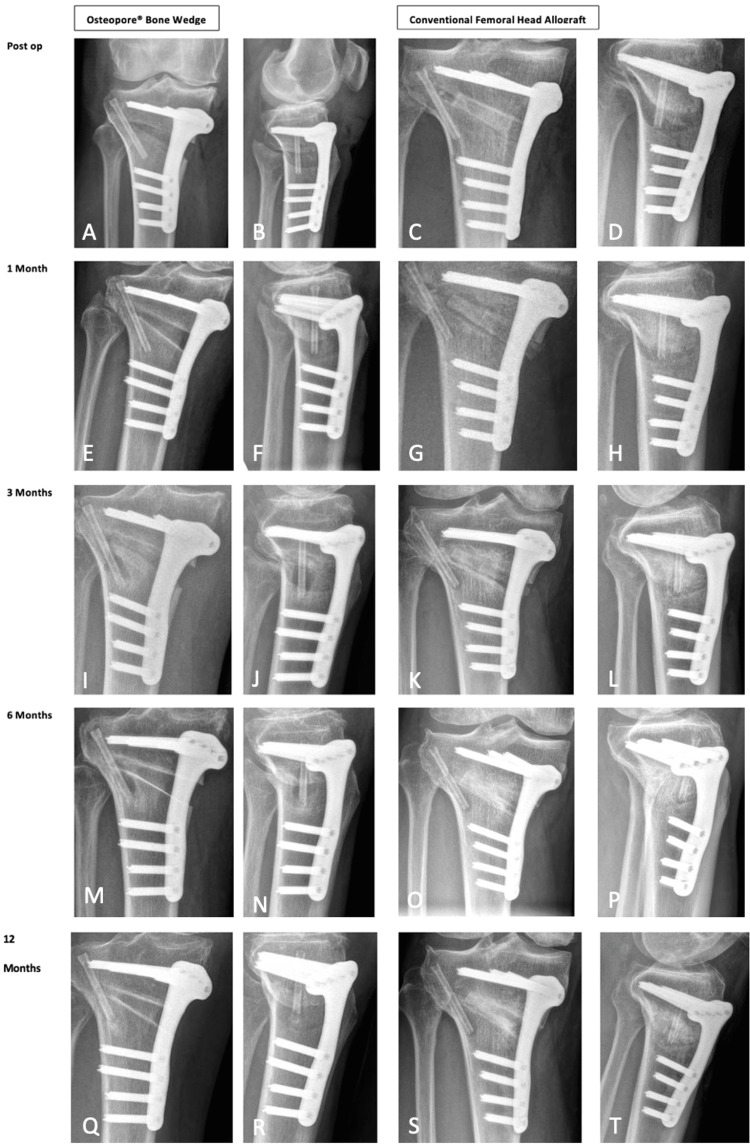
Postoperative radiographs comparing Osteopore® bone wedge and conventional femoral head allograft for up to 12 months. A: anteroposterior view of the Osteopore® bone wedge immediately postoperatively; B: lateral view of the Osteopore® bone wedge immediately postoperatively; C: anteroposterior view of the conventional femoral head allograft immediately postoperatively; D: lateral view of the conventional femoral head allograft immediately postoperatively; E: anteroposterior view of the Osteopore® bone wedge one month postoperatively; F: lateral view of the Osteopore® bone wedge one month postoperatively; G: anteroposterior view of the conventional femoral head allograft one month postoperatively; H: lateral view of the conventional femoral head allograft one month postoperatively; I: anteroposterior view of the Osteopore® bone wedge three months postoperatively; J: lateral view of the Osteopore® bone wedge three months postoperatively; K: anteroposterior view of the conventional femoral head allograft three months postoperatively; L: lateral view of the conventional femoral head allograft three months postoperatively; M: anteroposterior view of the Osteopore® bone wedge six months postoperatively; N: lateral view of the Osteopore® bone wedge six months postoperatively; O: anteroposterior view of the conventional femoral head allograft six months postoperatively; P: lateral view of the conventional femoral head allograft six months postoperatively; Q: anteroposterior view of the Osteopore® bone wedge 12 months postoperatively; R: lateral view of the Osteopore® bone wedge 12 months postoperatively; S: AP view of the conventional femoral head allograft 12 months postoperatively; T: lateral view of the conventional femoral head allograft 12 months postoperatively.
